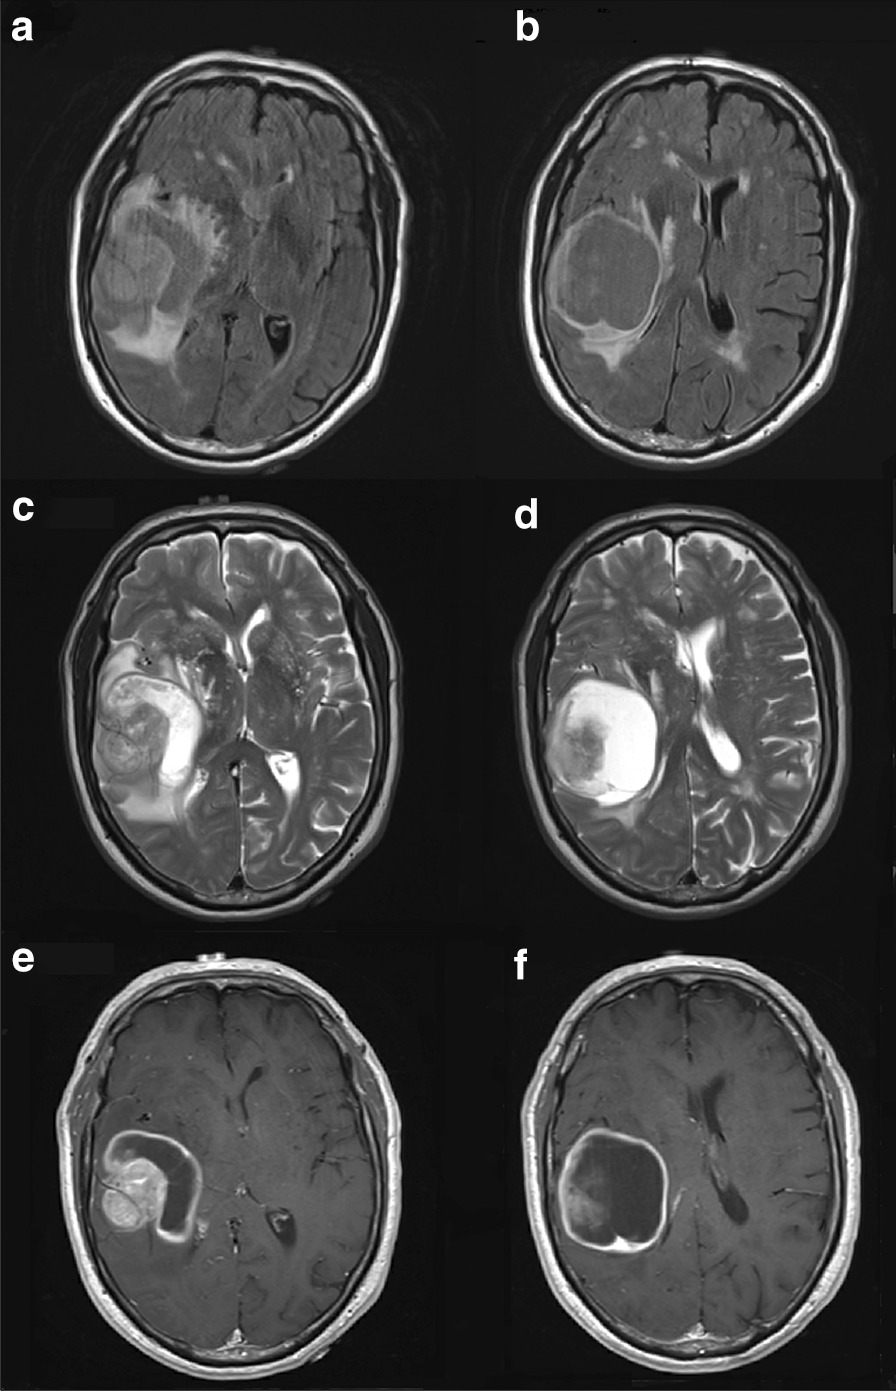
a, b Fluid-attenuated inversion recovery (FLAIR) and c, d T2-weighted imaging show increased T2 signal involving the right temporal lobe and right frontal lobe and no evidence of hemorrhage. A 9 mm right-to-left midline shift is shown with early right uncal herniation, effacement of the right lateral ventricle, and dilatation of the temporal horn of the right lateral ventricle. The hyperintense areas surrounding the lateral ventricles (a, b) are likely representative of leukoaraiosis rather than tumor lesions. e, f T1 imaging shows a 6.3 cm × 5.5 cm × 4.8 cm ring-enhancing heterogeneous cystic mass in the right frontotemporal region with a 3.5 cm × 3.0 cm avidly enhancing mural nodule with diffusion restriction (diffusion imaging not shown) on the lateral aspect of the mass
